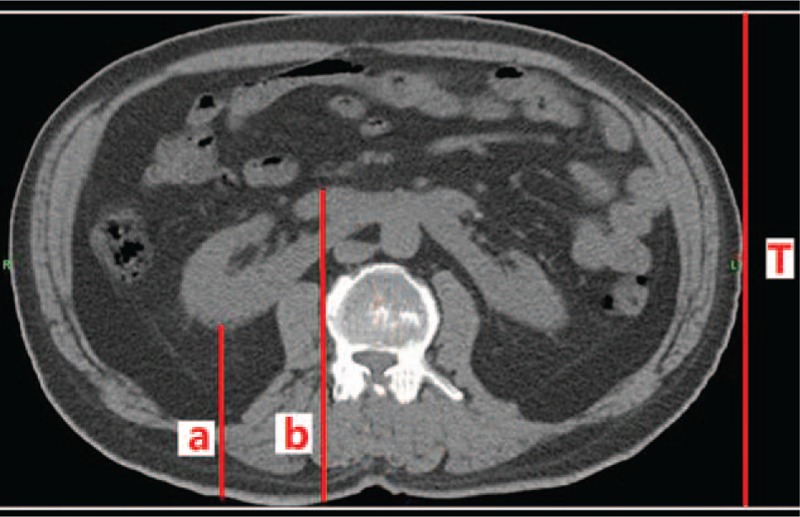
CT scan showing skin to anterior and posterior renal surfaces at the level of the renal hilum. Renal depth was determined by averaging the anterior and posterior depths at the renal hilum: renal depth = (a + b)/2; T is total thickness of the body at the level of the kidneys.
